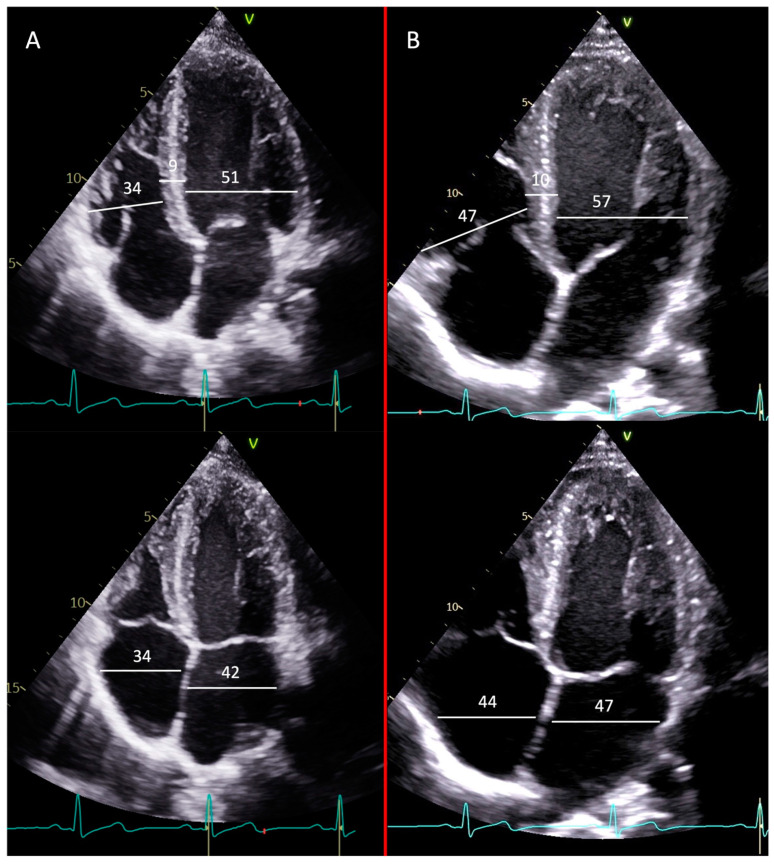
Transthoracic echocardiogram, apical four chamber view; upper figures: diastole; lower figures: systole. Panel (A): weightlifter. Panel (B): judoka. The athlete practicing judo presented larger diameters for both the ventricle and atria.
